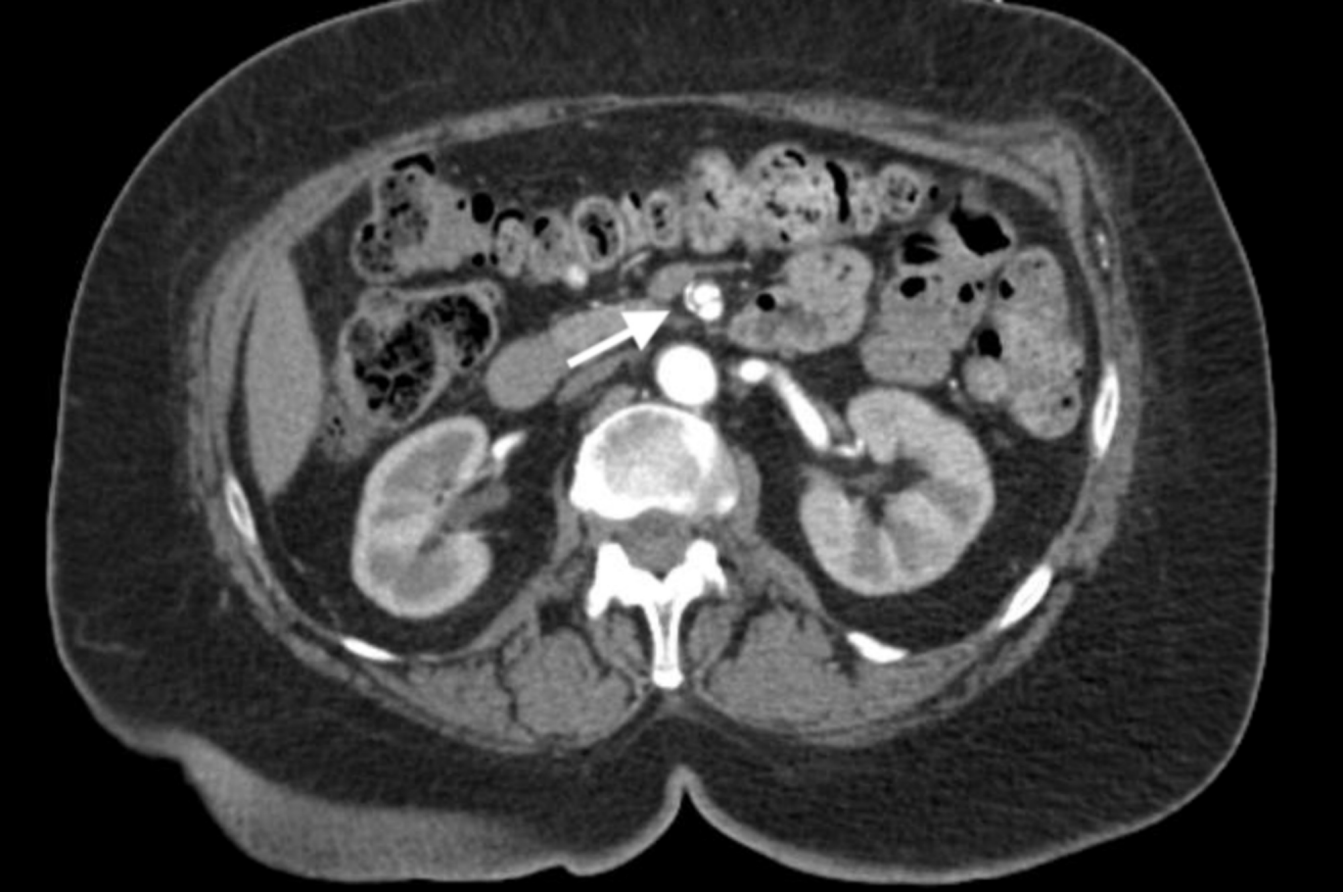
Cross-sectional view of isolated superior mesenteric artery dissection (indicated by arrow) on a CT angiogram.
